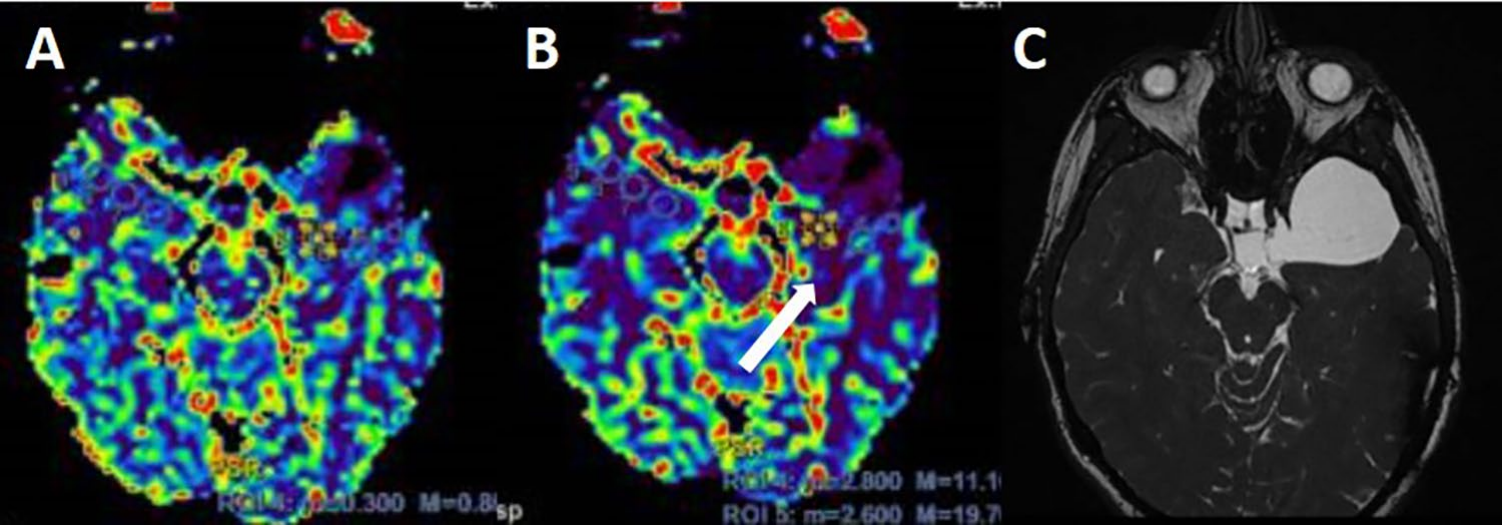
Perfusion MRI (A, B) showing a decrease of the signal in the temporal brain surrounding the SAC. The MRI with FIESTA sequences points out no communication of the cyst with the basal cisterns and, at the same time, enough room for a surgical fenestration (C)
